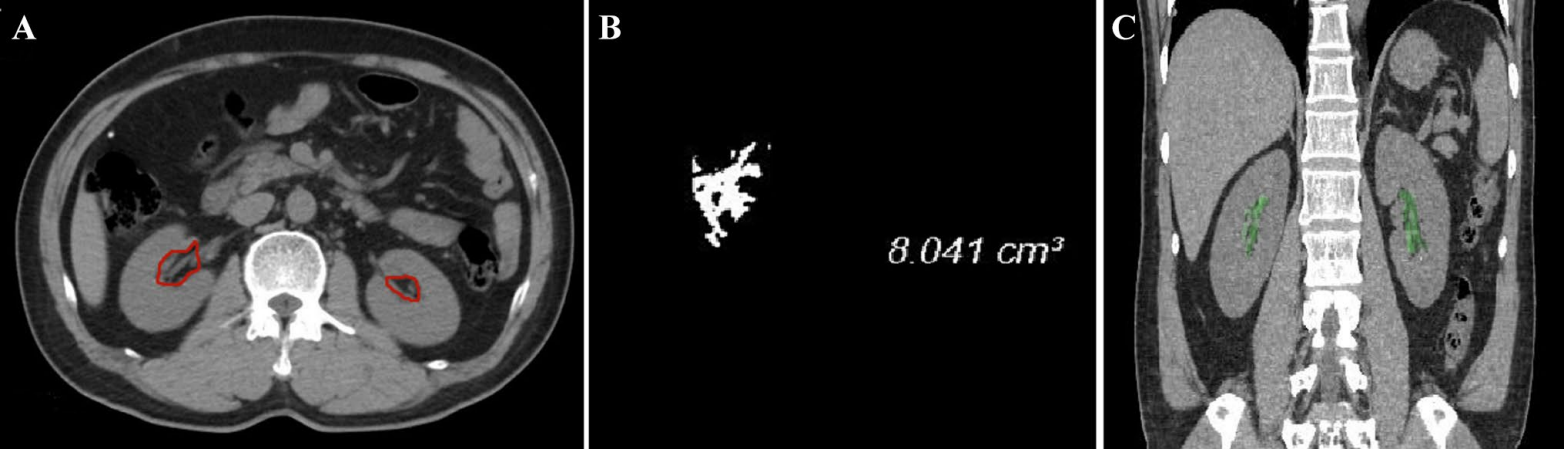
Abdominal computed tomography (CT) scans demonstrating the renal sinus fat measurement technique. a Example of a single CT slice selected for renal sinus fat measurement. The reader manually traced within the border of the kidney. b One measurement was taken in the left renal sinus. c The renal sinus is traced in this figure, highlighting the visualization of fat tissue observed in this region of interest
